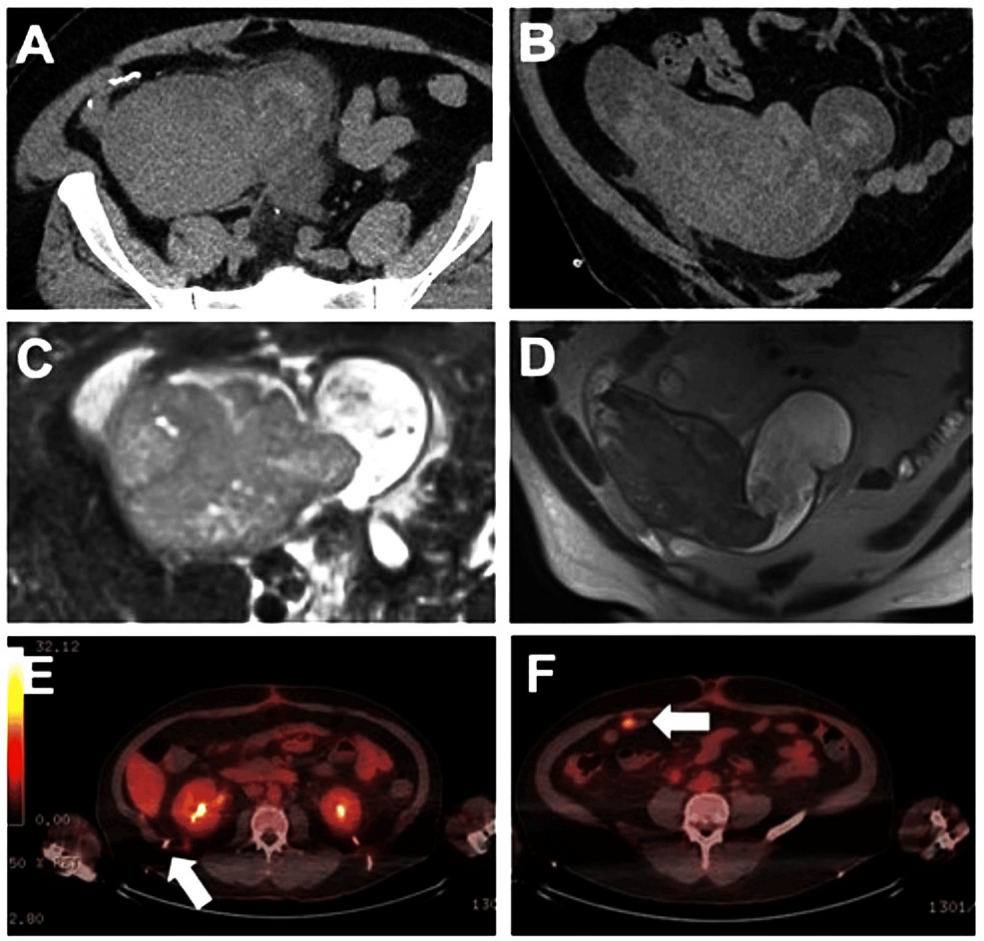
Cross-sectional imaging Representative cross-sectional imaging with CT (A&B), and MRI (C&D) with a mass in the ileal conduit. Arrows in panels E and F demonstrate, 19F FDG PET images with right hypermetabolic retroperitoneal (E), and omental soft-tissue implant (F). Interventional radiology-guided biopsy was performed on the retroperitoneal implant depicted in E.
